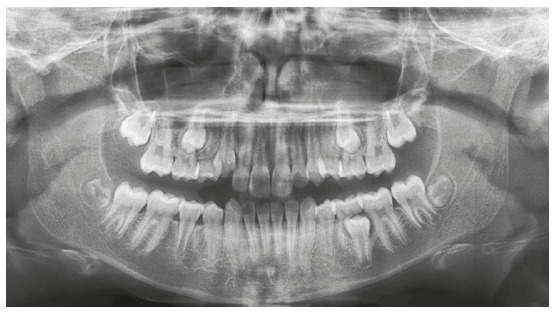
Case 2: Baseline panoramic radiograph.
